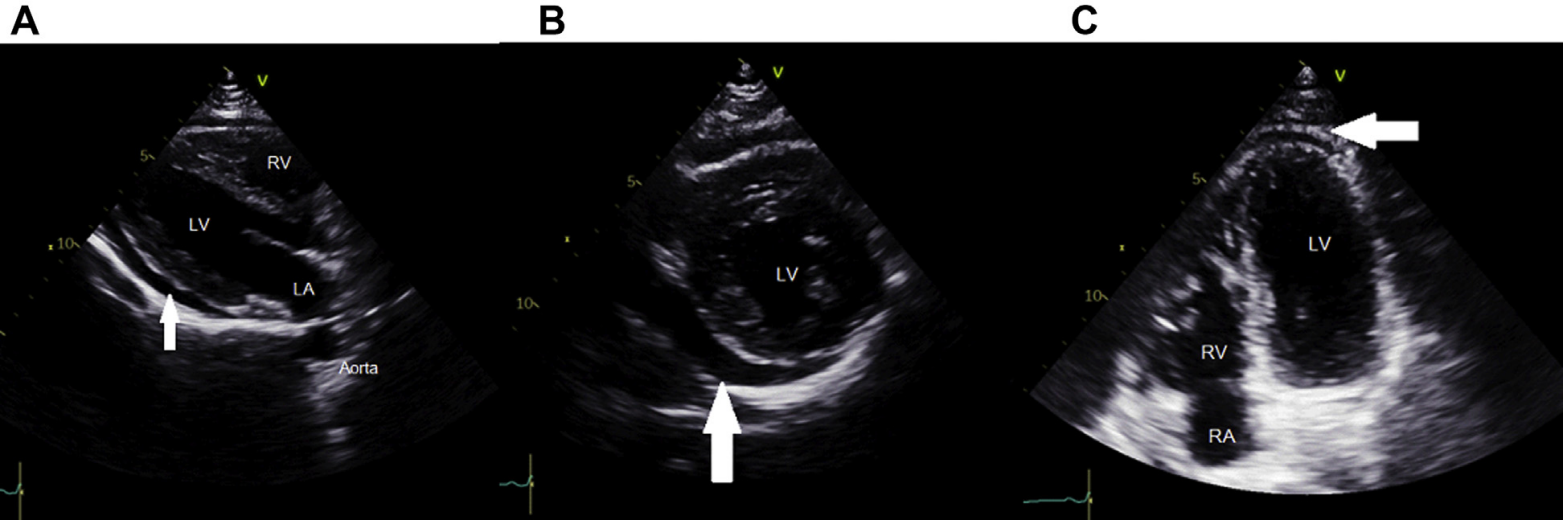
Initial Echocardiography (A) Parasternal long-axis, (B) parasternal short-axis, and (C) off-axis apical views on transthoracic echocardiography showing a small pericardial effusion (arrows). LA = left atrium; LV = left ventricle; RA = right atrium; RV = right ventricle.
